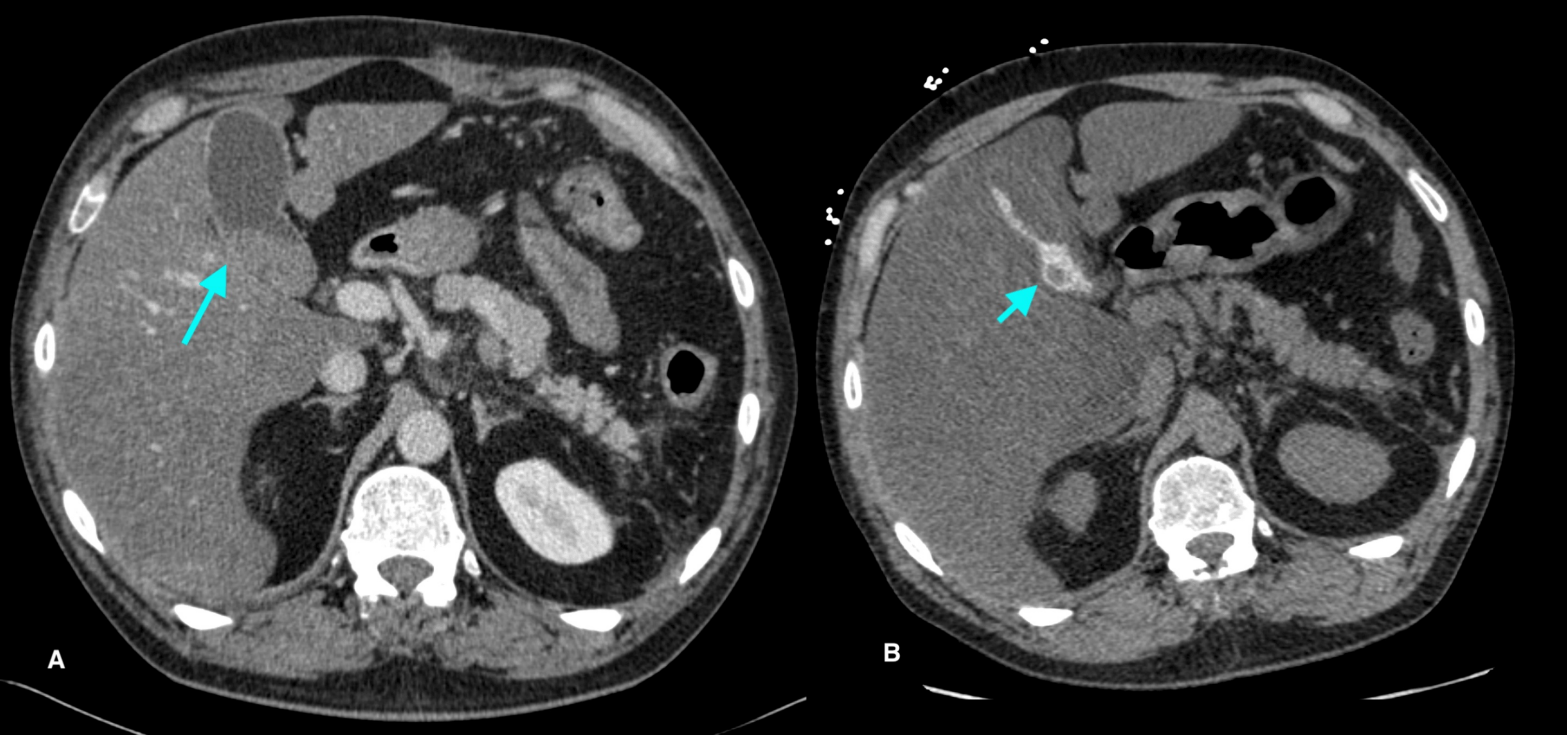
Computed tomography. A. Portal venous phase CT shows layering sludge and debris in the gallbladder at initial presentation in 2017 (A, long arrow). B. Subsequent un-enhanced CT performed one year later (2018) shows a dramatic change with lobulated, layering calcification along the gallbladder wall (B, short arrow).
